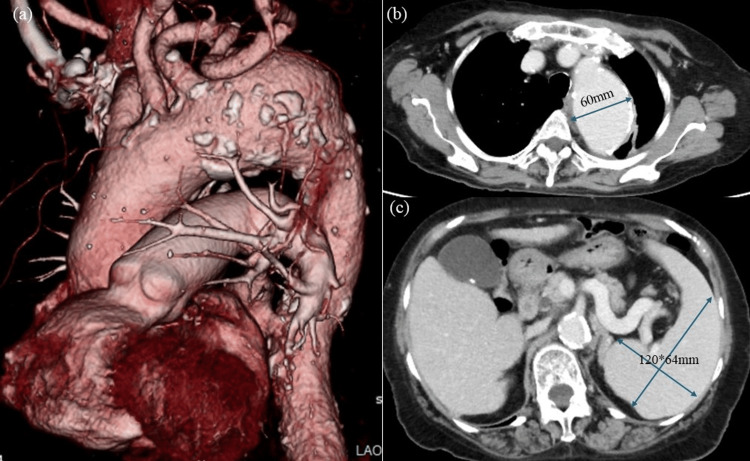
Preoperative computed tomography images (a) and (b) show the distal arch aneurysm with a diameter of 60 mm. The left subclavian artery was derived from the aneurysm. (c) shows splenomegaly (120 x 64 mm).
